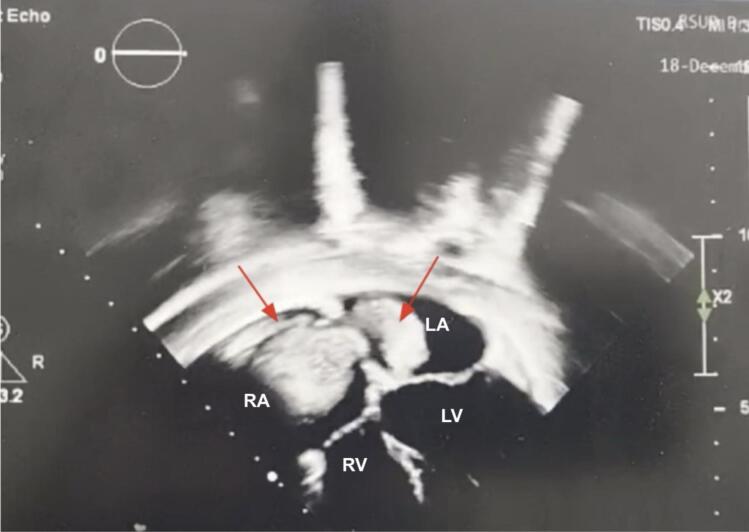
Preoperative echocardiography showing mass (red arrows) filling RA and LA which connected via atrial septum. LA (left atrium); RA (right Atrium); LV (left ventricle); RV (right ventricle).
